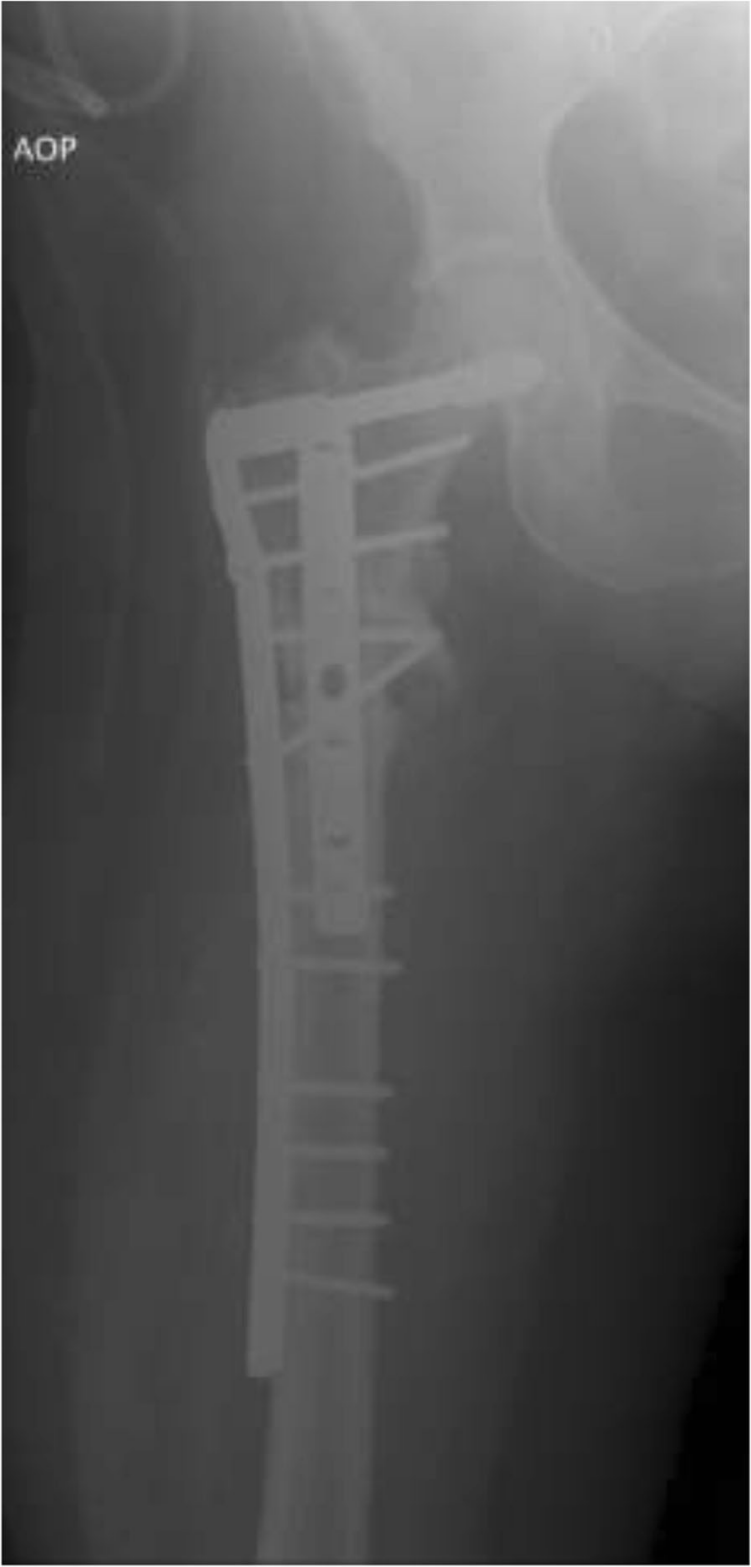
X-ray after implant removal, nonunion debridement, restoration of the CCD (131°) and ORIF utilizing a DCS with an additional LCDCP
